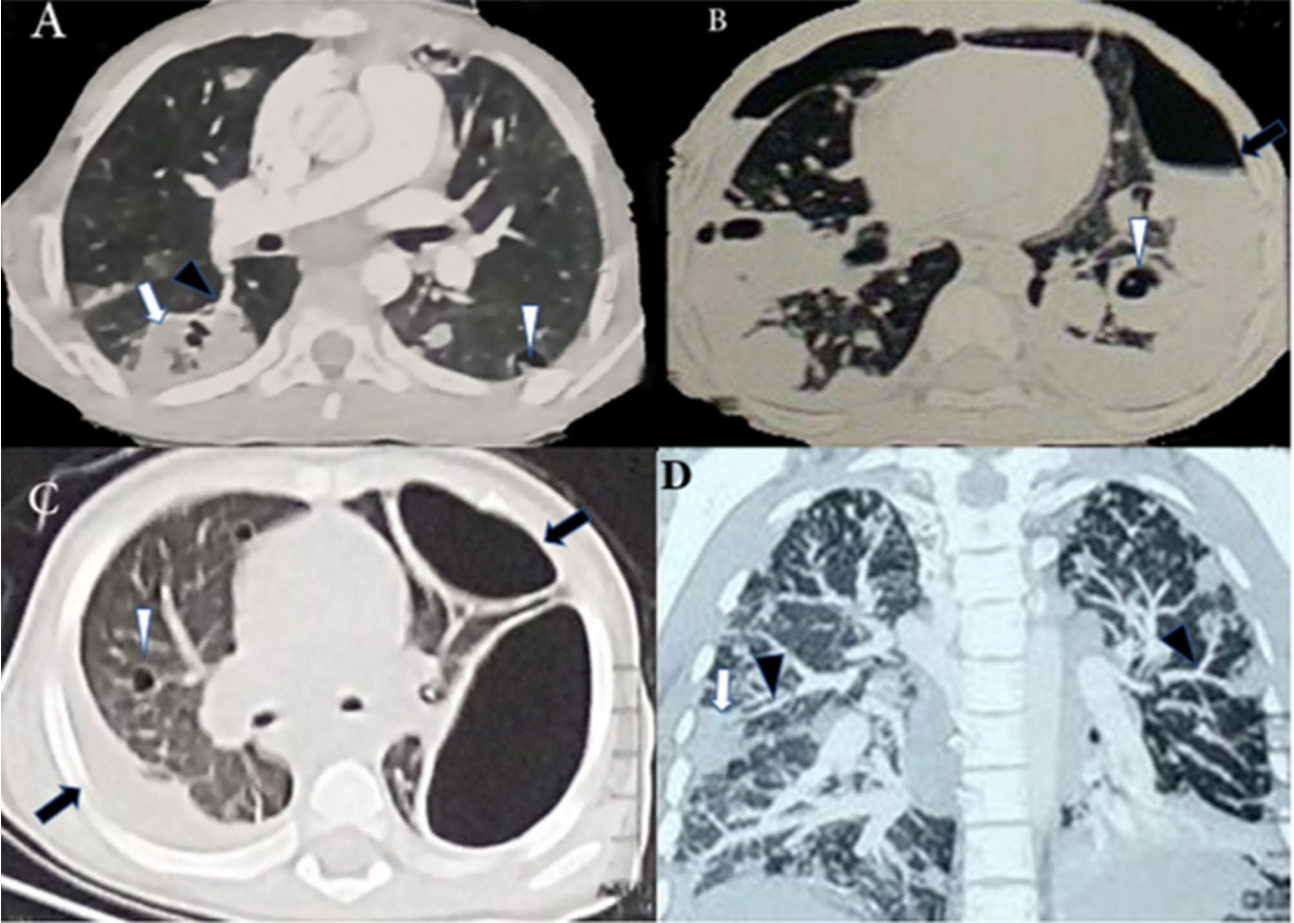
Chest CT revealed (A) right side peripheral wedge-shaped lesions (white arrow), feeding vessel sign (black arrowhead), and left side peripheral cavity (white arrowhead). (B) Bilateral pneumothorax (black arrow), and bilateral cavitary lesions (white arrowhead). (C) Left side encysted pneumothorax (black arrow), right multiple cavitary lesions (white arrowhead), and right side pleural effusion (black arrow). (D) Bilateral multiple nodular lesions (white arrow), and feeding vessel sign (black arrowhead)
